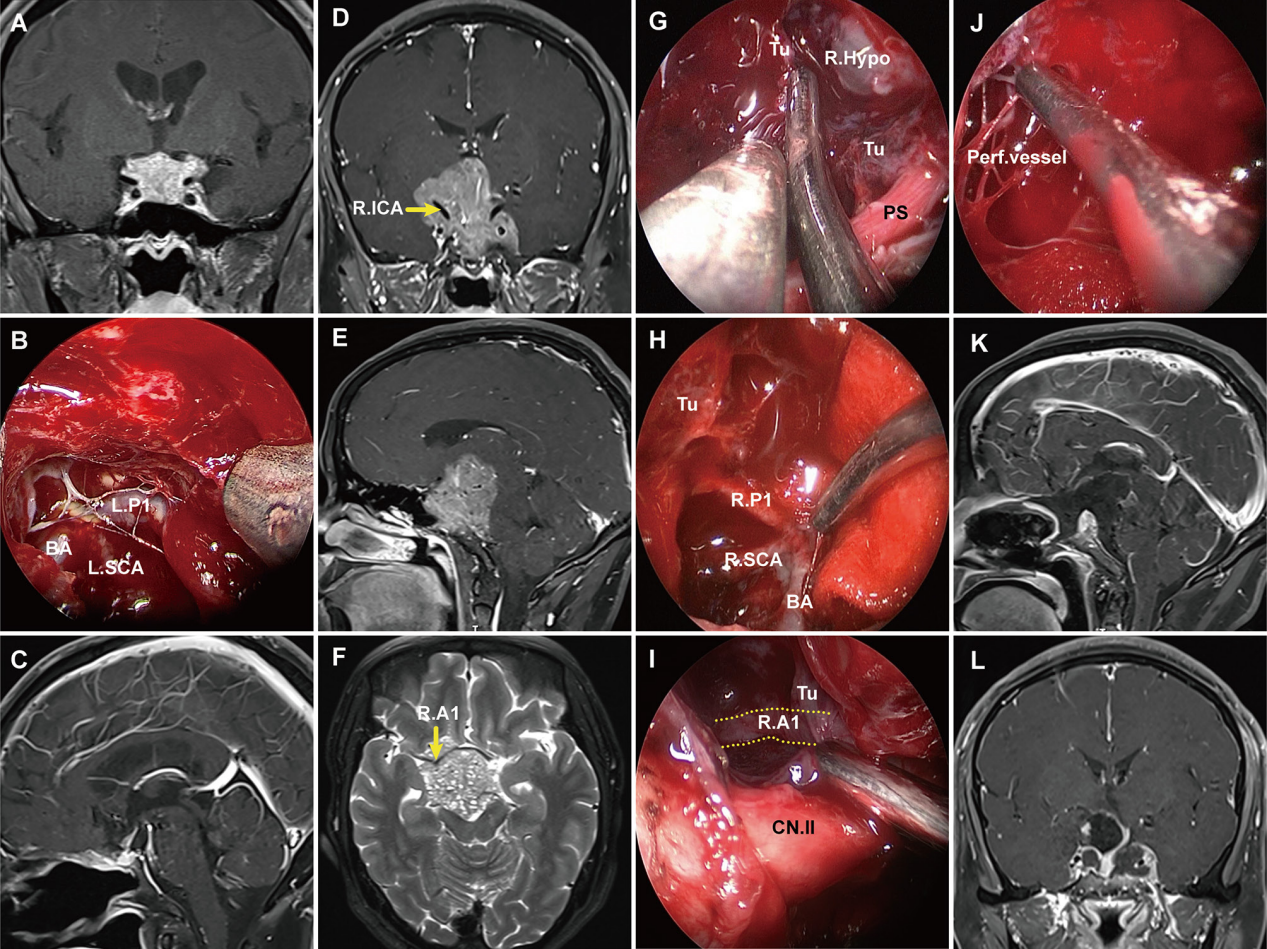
Pre- and postoperative magnetic resonance imaging (MRI) demonstrating two cases of grade 2 pituitary adenoma. (A) A grade 2 PA that extended to the suprasellar region and grew along with the suprasellar cistern. (B) Endoscopic views showing the tumor entered into the arachnoid spaces and encased vital neurovascular structures. (C) postoperative MRI showing gross total resection. (D–F) Preoperative T1 contrast-enhanced images showing a grade 2 tumor significantly extended to the suprasellar and encircled vital neurovascular structures. (G–J) Endoscopic views showing the tumor entered into the suprasellar space and encased vital neurovascular structures. The tumor grew in grid-like structures formed by the perforating vessels (J). (K, L) Postoperative T1 contrast-enhanced MRI demonstrating subtotal resection of the suprasellar component of adenoma. PS, pituitary stalk; PG, pituitary gland; Tu, tumor; R. A1, right A1 segment of anterior cerebral artery; R. P1, right P1 segment of posterior cerebral artery; SCA, superior cerebellar artery; BA, basilar artery; R. Ht, right hypothalamus.
